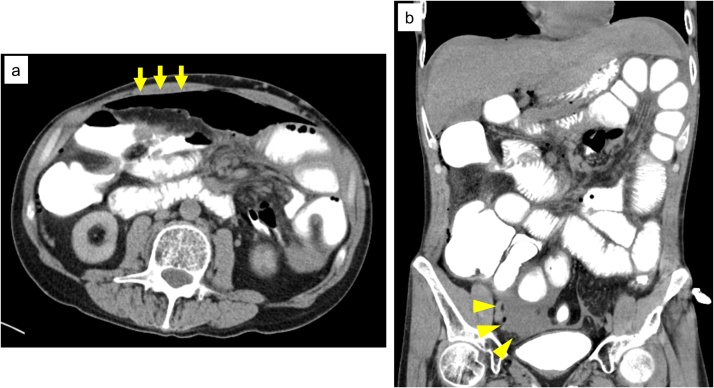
CT findings. CT showed intraperitoneal free air (a: yellow arrows) and a small amount of ascites (b: yellow arrowheads). Leakage of contrast agent into the intraperitoneal cavity was not observed.
